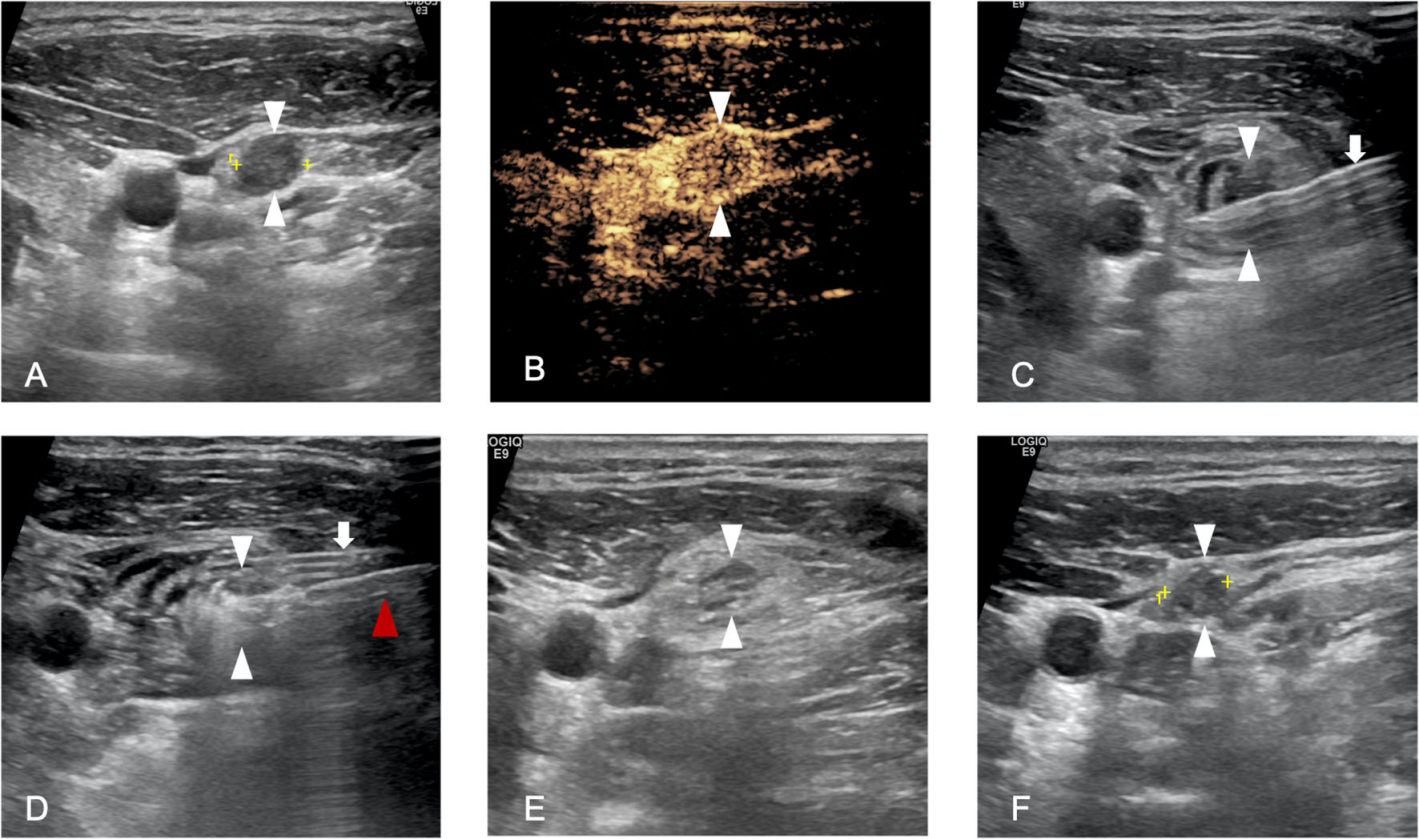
A 36-year-old man who underwent microwave ablation (MWA) of cervical lymph node metastasis (LNM). (A), Pre-MWA, B-mode ultrasonography (US) showed hypoechoic LN (white arrowhead); (B), Pre-MWA, the contrast-enhanced US (CEUS) showed uneven enhanced patterns (white arrowhead); (C), Hydrodissection fluid was injected surround the LNM (white arrowhead) by 18G PTC needle(white arrow); (D), Hyperechoic (white arrowhead) pattern in the LNM during MWA, and red arrowhead refers to MWA antenna; (F), One day post-MWA, hyperechoic ablated needle tract was showed inside the LNM (white arrowhead); (F), One month post-MWA, the size of LNM decreased compared to pre-MWA.
